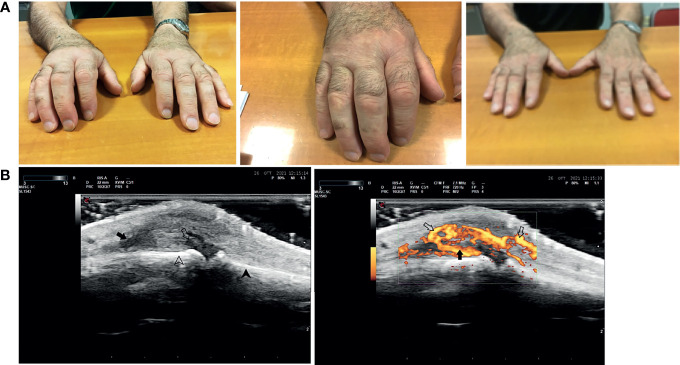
Rheumatoid arthritis-like clinical presentation closely correlated with anti-SARS-2 vaccination. The figure shows a representative case of a rheumatoid arthritis-like clinical presentation in a patient after SARS-COV2 vaccination. Panel (A) From left to right: acute phase of the disease, a particular of the right hand, and clinical examination after 4 weeks of corticosteroid therapy. Panel (B) Ultrasound doppler examination of the 3rd metacarpal-phalangeal joint of the right hand (from left to right): Grayscale US, Joint capsule (*arrow*) of MCF III dilated by synovial proliferation (*open arrow*) grade III (gs) according to OMERACT criteria. Below are shown the surfaces of metacarpal bone (arrowhead) and proximal phalanx (open arrowhead); Power Doppler Same scan of Figure 1 with PD showing severly active synovitis (*arrow*) of grade III (pd) according to OMERACT criteria With PD signal becames evident an associated paratenonitis of third digit extensor tendons (open arrows).
